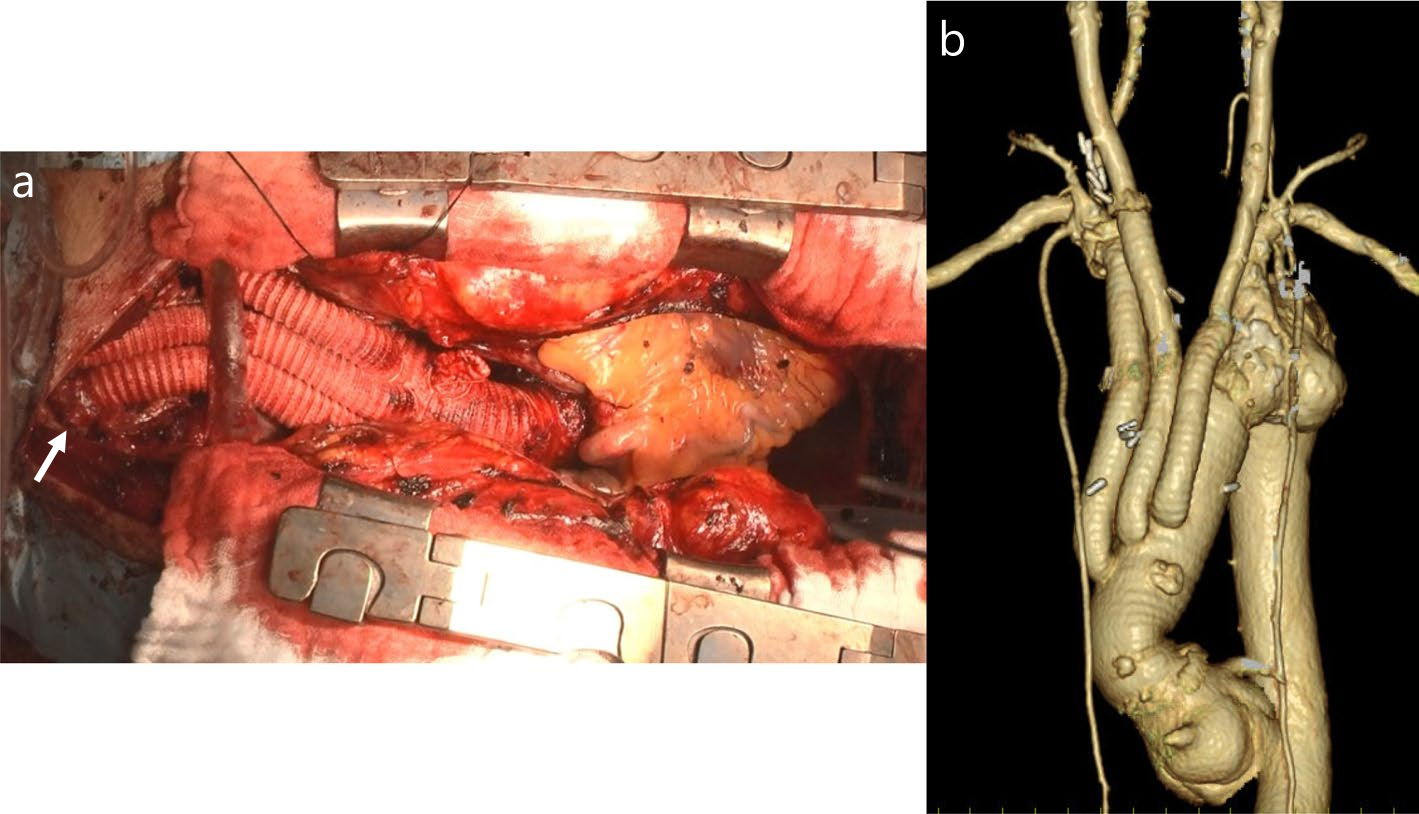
a Intraoperative image showing the right subclavian artery anastomosis site (arrow head). b Postoperative three-dimensional computed tomography image of the initial open procedure
